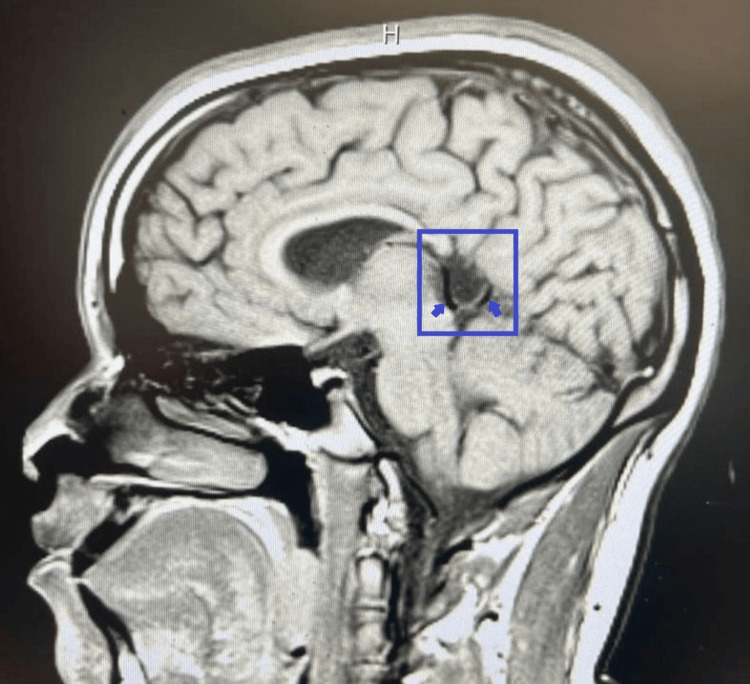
Sagittal T1-weighted MRI. Mid-sagittal T1-weighted MRI demonstrating absence of the splenium of the corpus callosum. A CSF-filled space is seen posterior to the third ventricle, communicating with it (blue rectangle). Arrows highlight the CSF-filled region.
